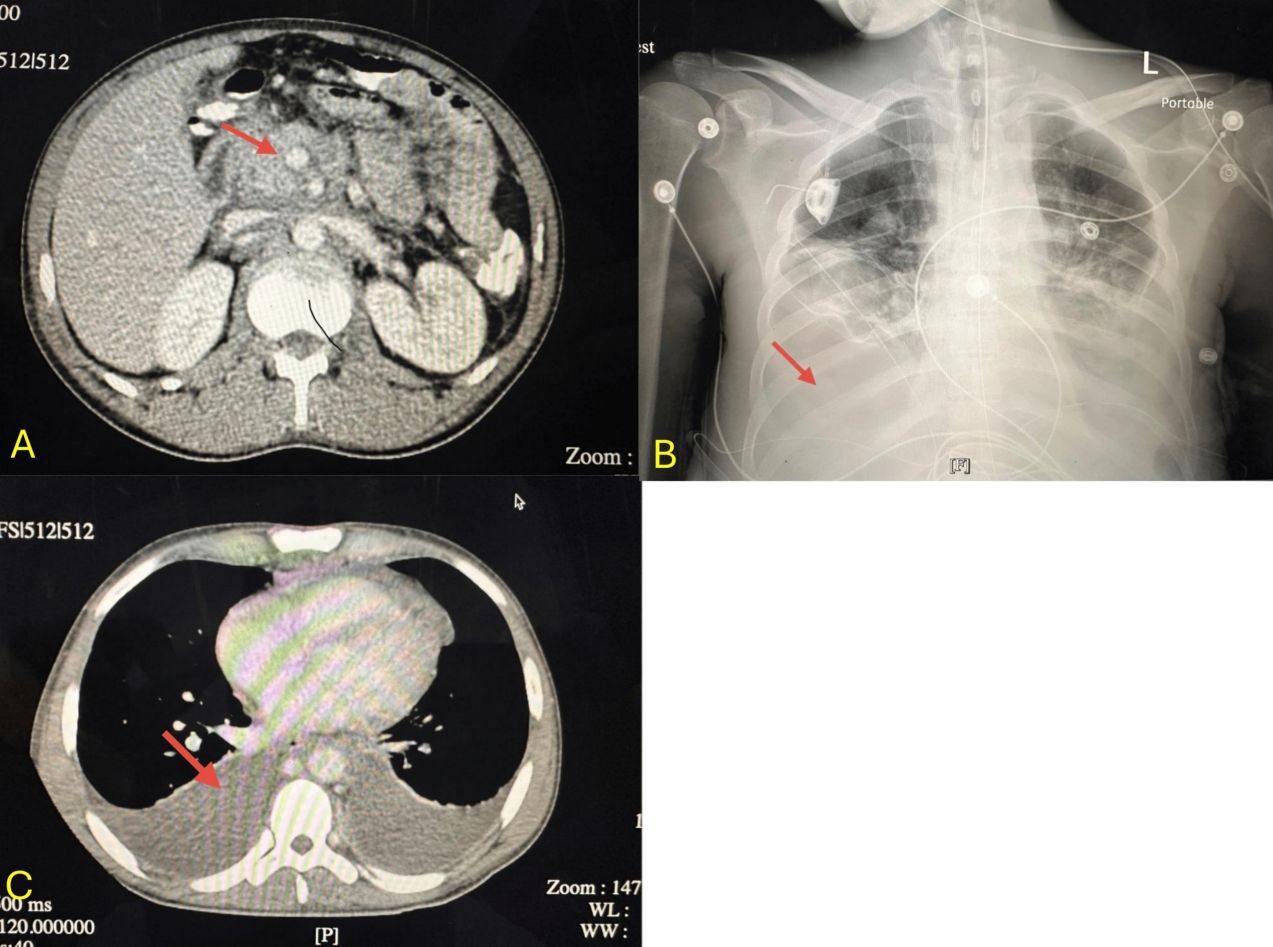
A: CT abdomen/pelvis showing lymphadenopathy. B: Chest X-ray showing bilateral pleural effusions. C: CT chest confirming pleural effusions.
